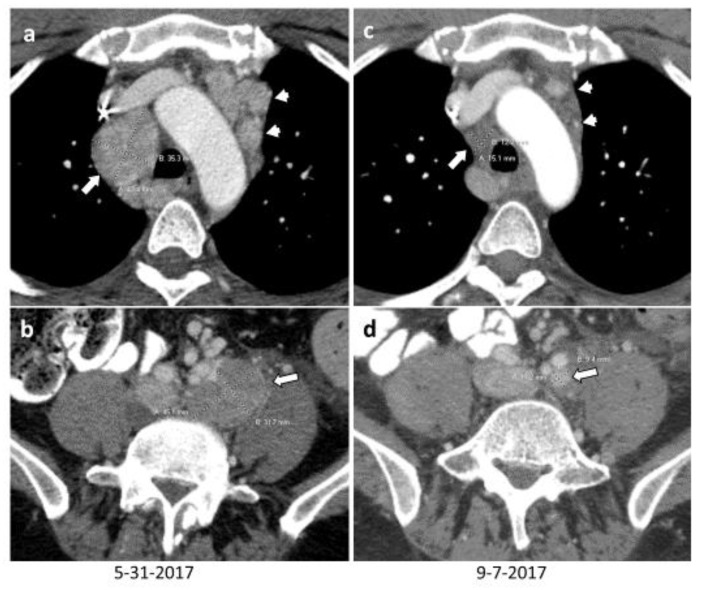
A 42-year-old man with metastatic paraganglioma underwent multiple surgery resection, post-surgical therapy with CVD × 6 months, cabozantinib × 8 months, then treatment with pembrolizumab. The pre-immunotherapy contrast enhanced CT (CECT) showed innumerable lymph node metastases in the chest, abdomen, and pelvis. At 2 months post initiation of immunotherapy, the lymph node metastases had significantly decreased by number and size (long and short arrows in images (a–d)). Representative axial CECT images ((a): pretherapy axial CECT of chest, (b): pretherapy axial CECT of pelvis, (c): post-therapy axial CECT of chest, (d): post-therapy axial CECT of pelvis) showed that the mediastinal (long and short arrows in (a,c)) and left common iliac (long arrow in (b,d)) lymph node metastases had significantly improved. The right paratracheal lymph node (long arrows in (a,c)) decreased from 4.3 × 3.5 cm to 1.5 × 1.2 cm, and the left common iliac lymph node (long arrows in (b,d)) decreased from 4.5 × 3.2 cm to 1.1 × 0.9 cm.
